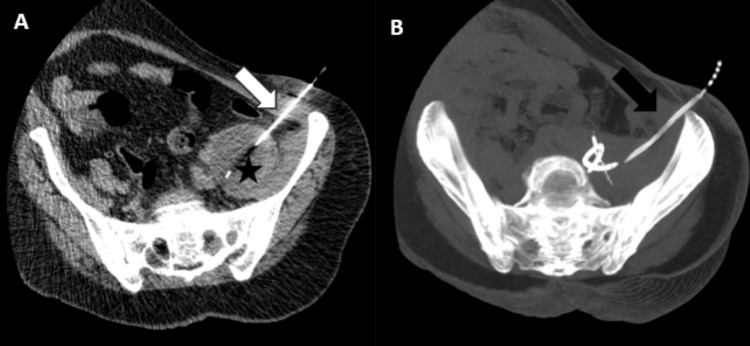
CT-guided catheter insertion in the left psoas abscess Axial (A) and axial maximum intensity projection (MIP) (B) images show a needle (white arrow), followed by a catheter (black arrow), within the left psoas abscess (*). CT, Computed Tomography
